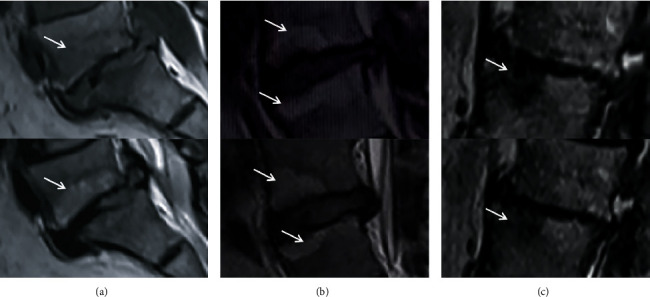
Modic classification for vertebral endplate changes (the patients pictured were not included in the study). (a) Type I: decreased signal on T1- and increased signal on T2-weighted images. (b) Type II: increased signals on both T1- and T2-weighted images. (c) Type III: decreased signals on both T1- and T2-weighted images.
